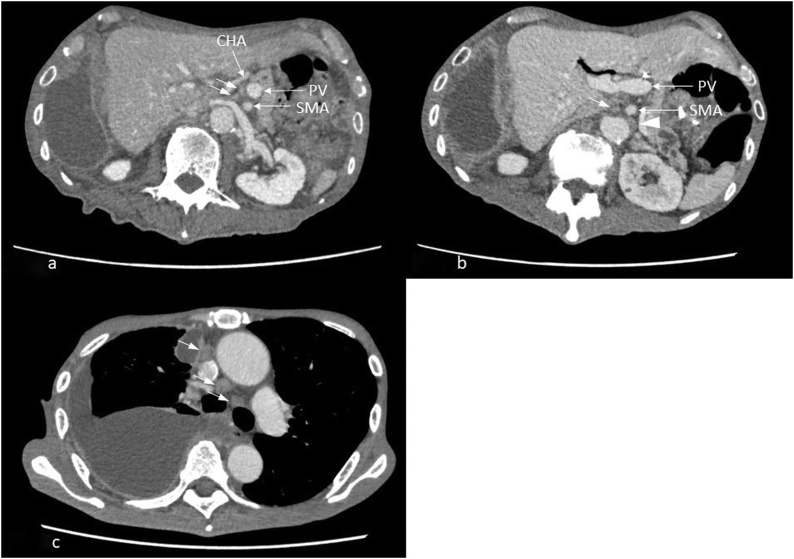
CT findings. a) Lymph node swelling was observed along the common hepatic artery (arrow). b) Superior mesenteric artery root (arrow) and paraaortic (arrowhead) lymph node swelling was noted. c) Right pleural effusion retention and swelling of multiple mediastinal lymph nodes were observed (arrow).
